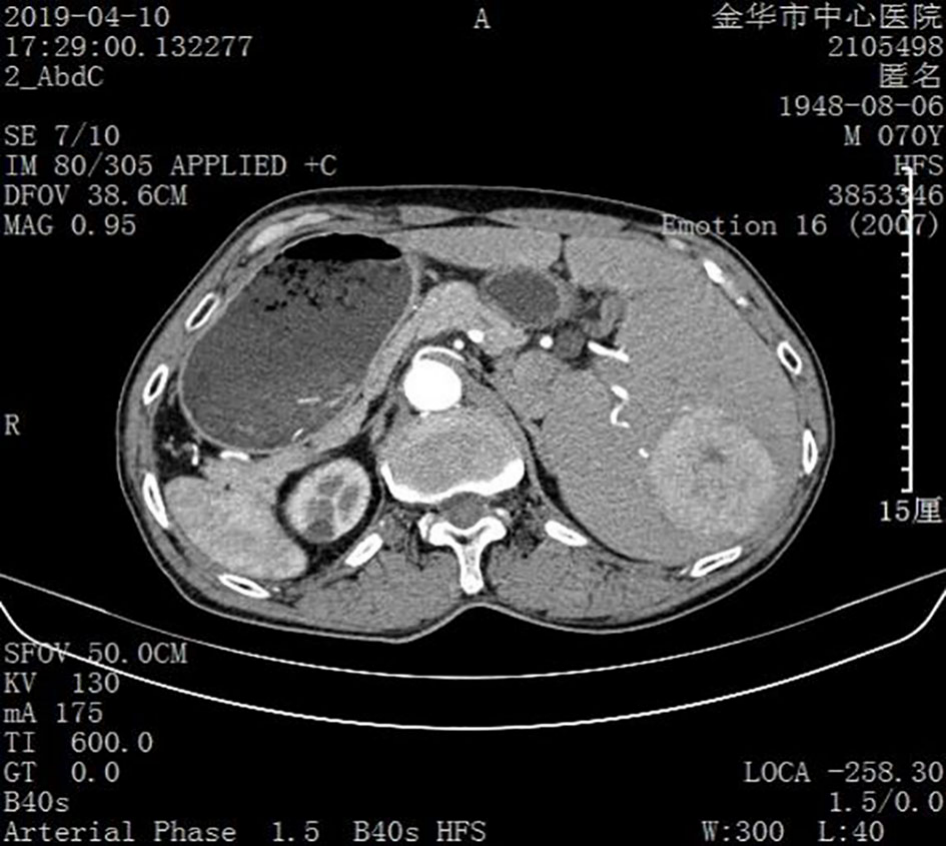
Image obtained using enhanced computed tomography. The liver and tumor are located in the left abdominal cavity.
